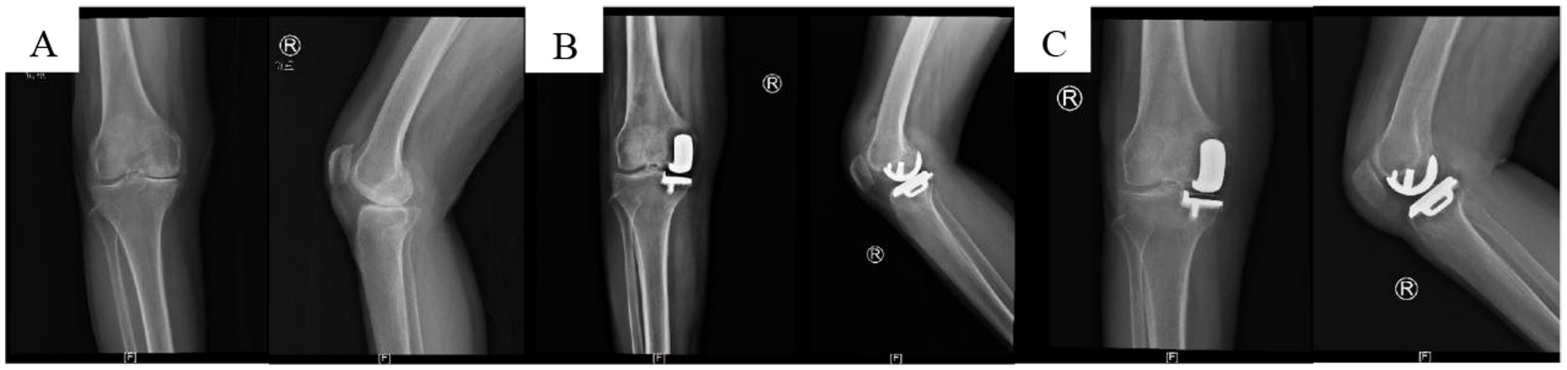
X-ray images of anterior medial knee OA before and after UKA surgery. (A) Preoperative image; (B) postoperative 2-day image; (C) postoperative 3-month image.
